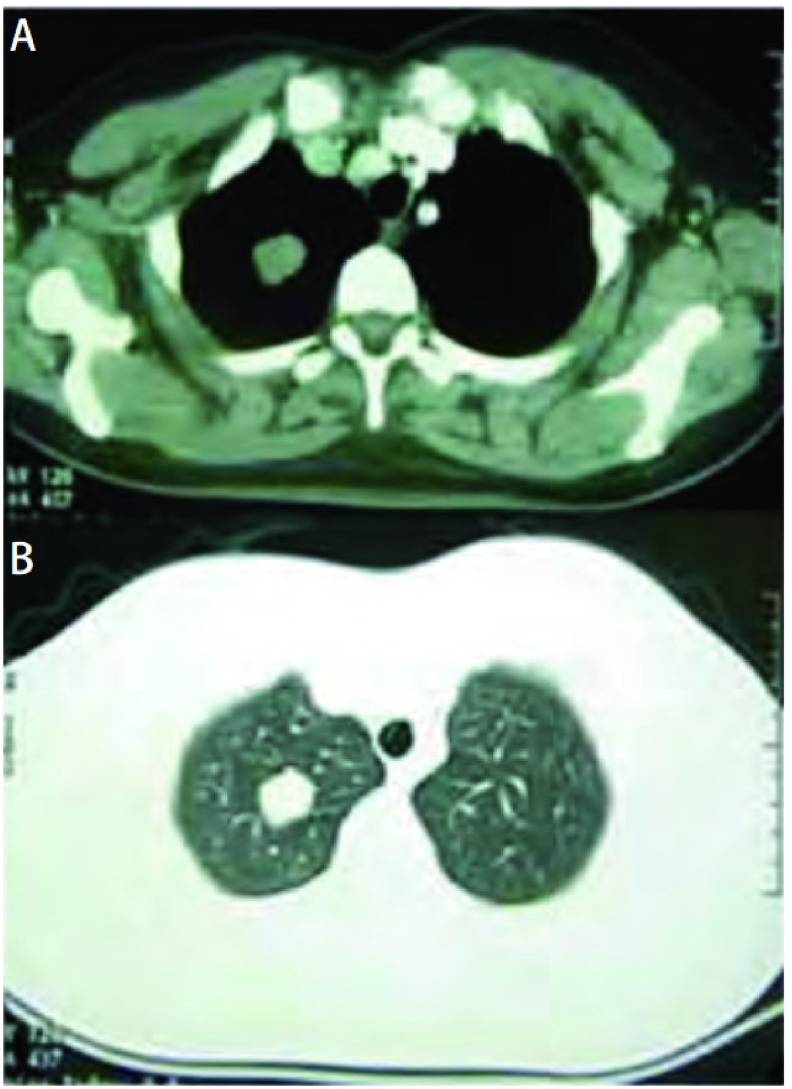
患者女性，35岁，体检发现右肺上叶肿物。CT示类圆形实性肿物，大小为20 mm×17 mm，密度均匀，边缘基本光滑，其内可见钙化斑。A：纵隔窗；B：肺窗。 Female patient, 35 years old, tumor was founded in right upper lobe of lung by medical examination. CT shows 20 mm×17 mm spherical solid mass, uniform density, boundary smooth and calcification within. A: mediastinal window; B: lung window.
